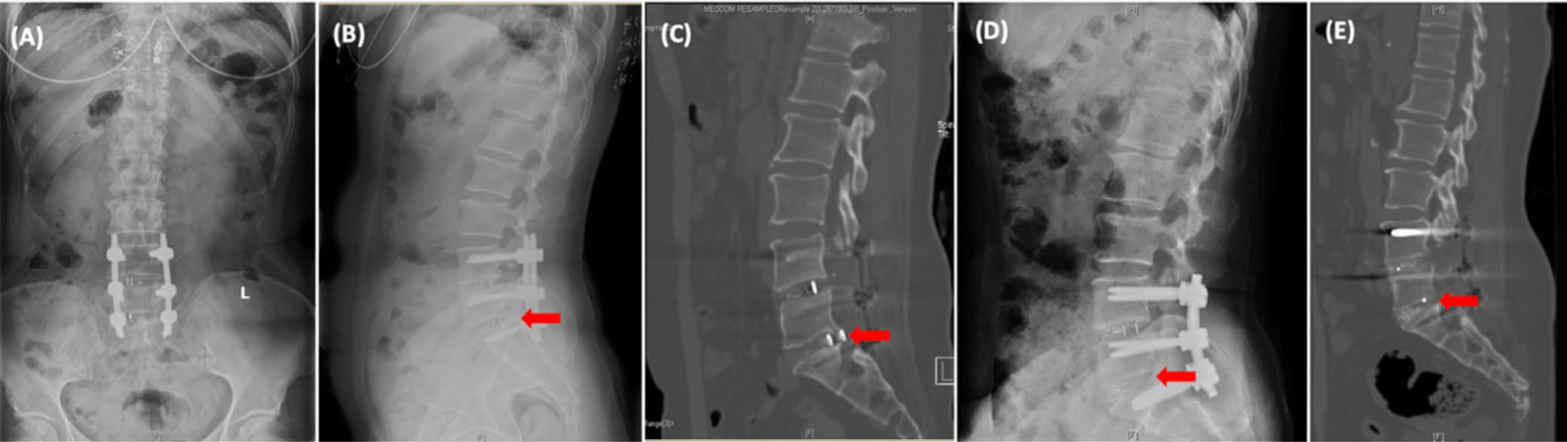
Images before and after revision surgery for a 53‐year‐old female patient. (A) Anteroposterior radiogram with the CR at L5/S1 before surgery. (B) Lateral radiogram with the CR at L5/S1 before revision surgery. (C) Sagittal reconstruction CT with the CR at L5/S1 before surgery. (D) Lateral radiogram after the revision surgery. The loose screws and cage were removed and replaced with larger ones, after which autologous bone grafts were re‐implanted for intervertebral fusion. (E) After the revision surgery, the final sagittal reconstruction CT at L5/S1 level showed that the patient's symptoms disappeared, and the CT indicated a successful fusion. The red arrow shows the posterior edge of the cage.
